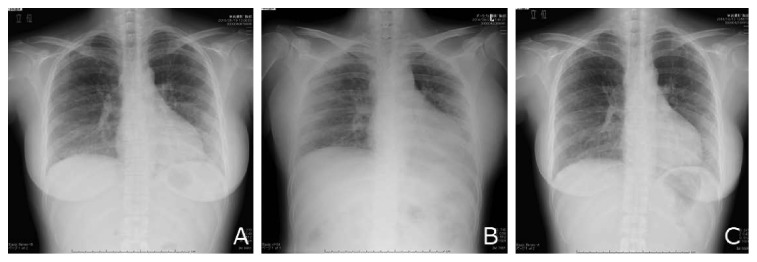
(a) Chest radiography on admission showing bilateral ground-glass opacity, (b) discharge radiography showing improvement of ground-glass opacity, and (c) postpartum radiography showing more improvement.
